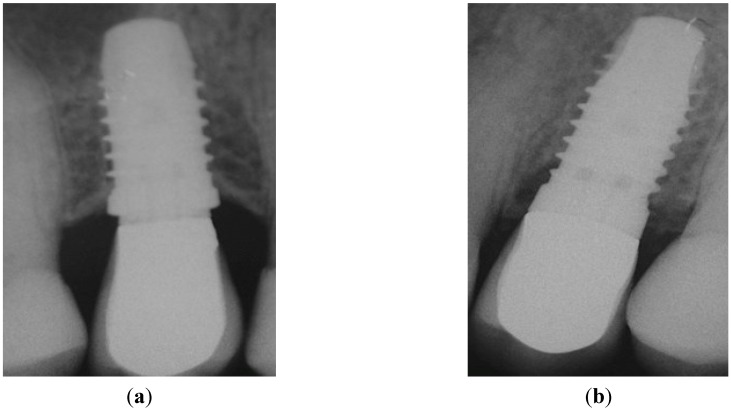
Peri-apical X-rays at one-year follow-up. (a) Position 15; (b) Position 25.
